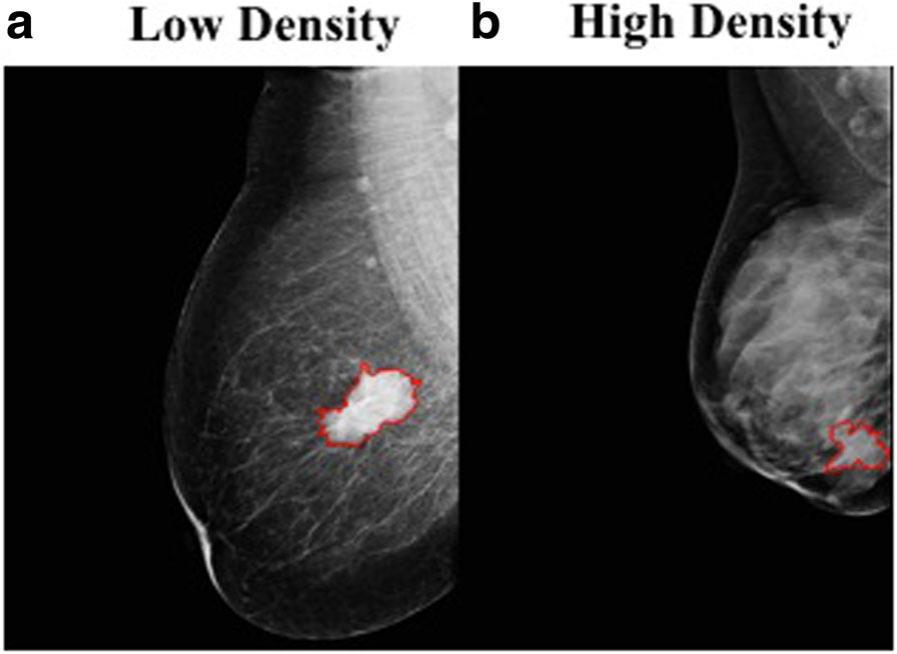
Exemplars of target-present images. The *red outline* depicts the mass (and did not appear in the actual stimuli). a Low-density breast that contains predominately fatty tissue, which is radio-translucent or black/grey. The higher contrast mass is easily seen. b High-density breast that contains normal fibroglandular tissue resulting in a more difficult search. The X-ray beam is attenuated by this tissue and appears radio-opaque or white on a mammogram
